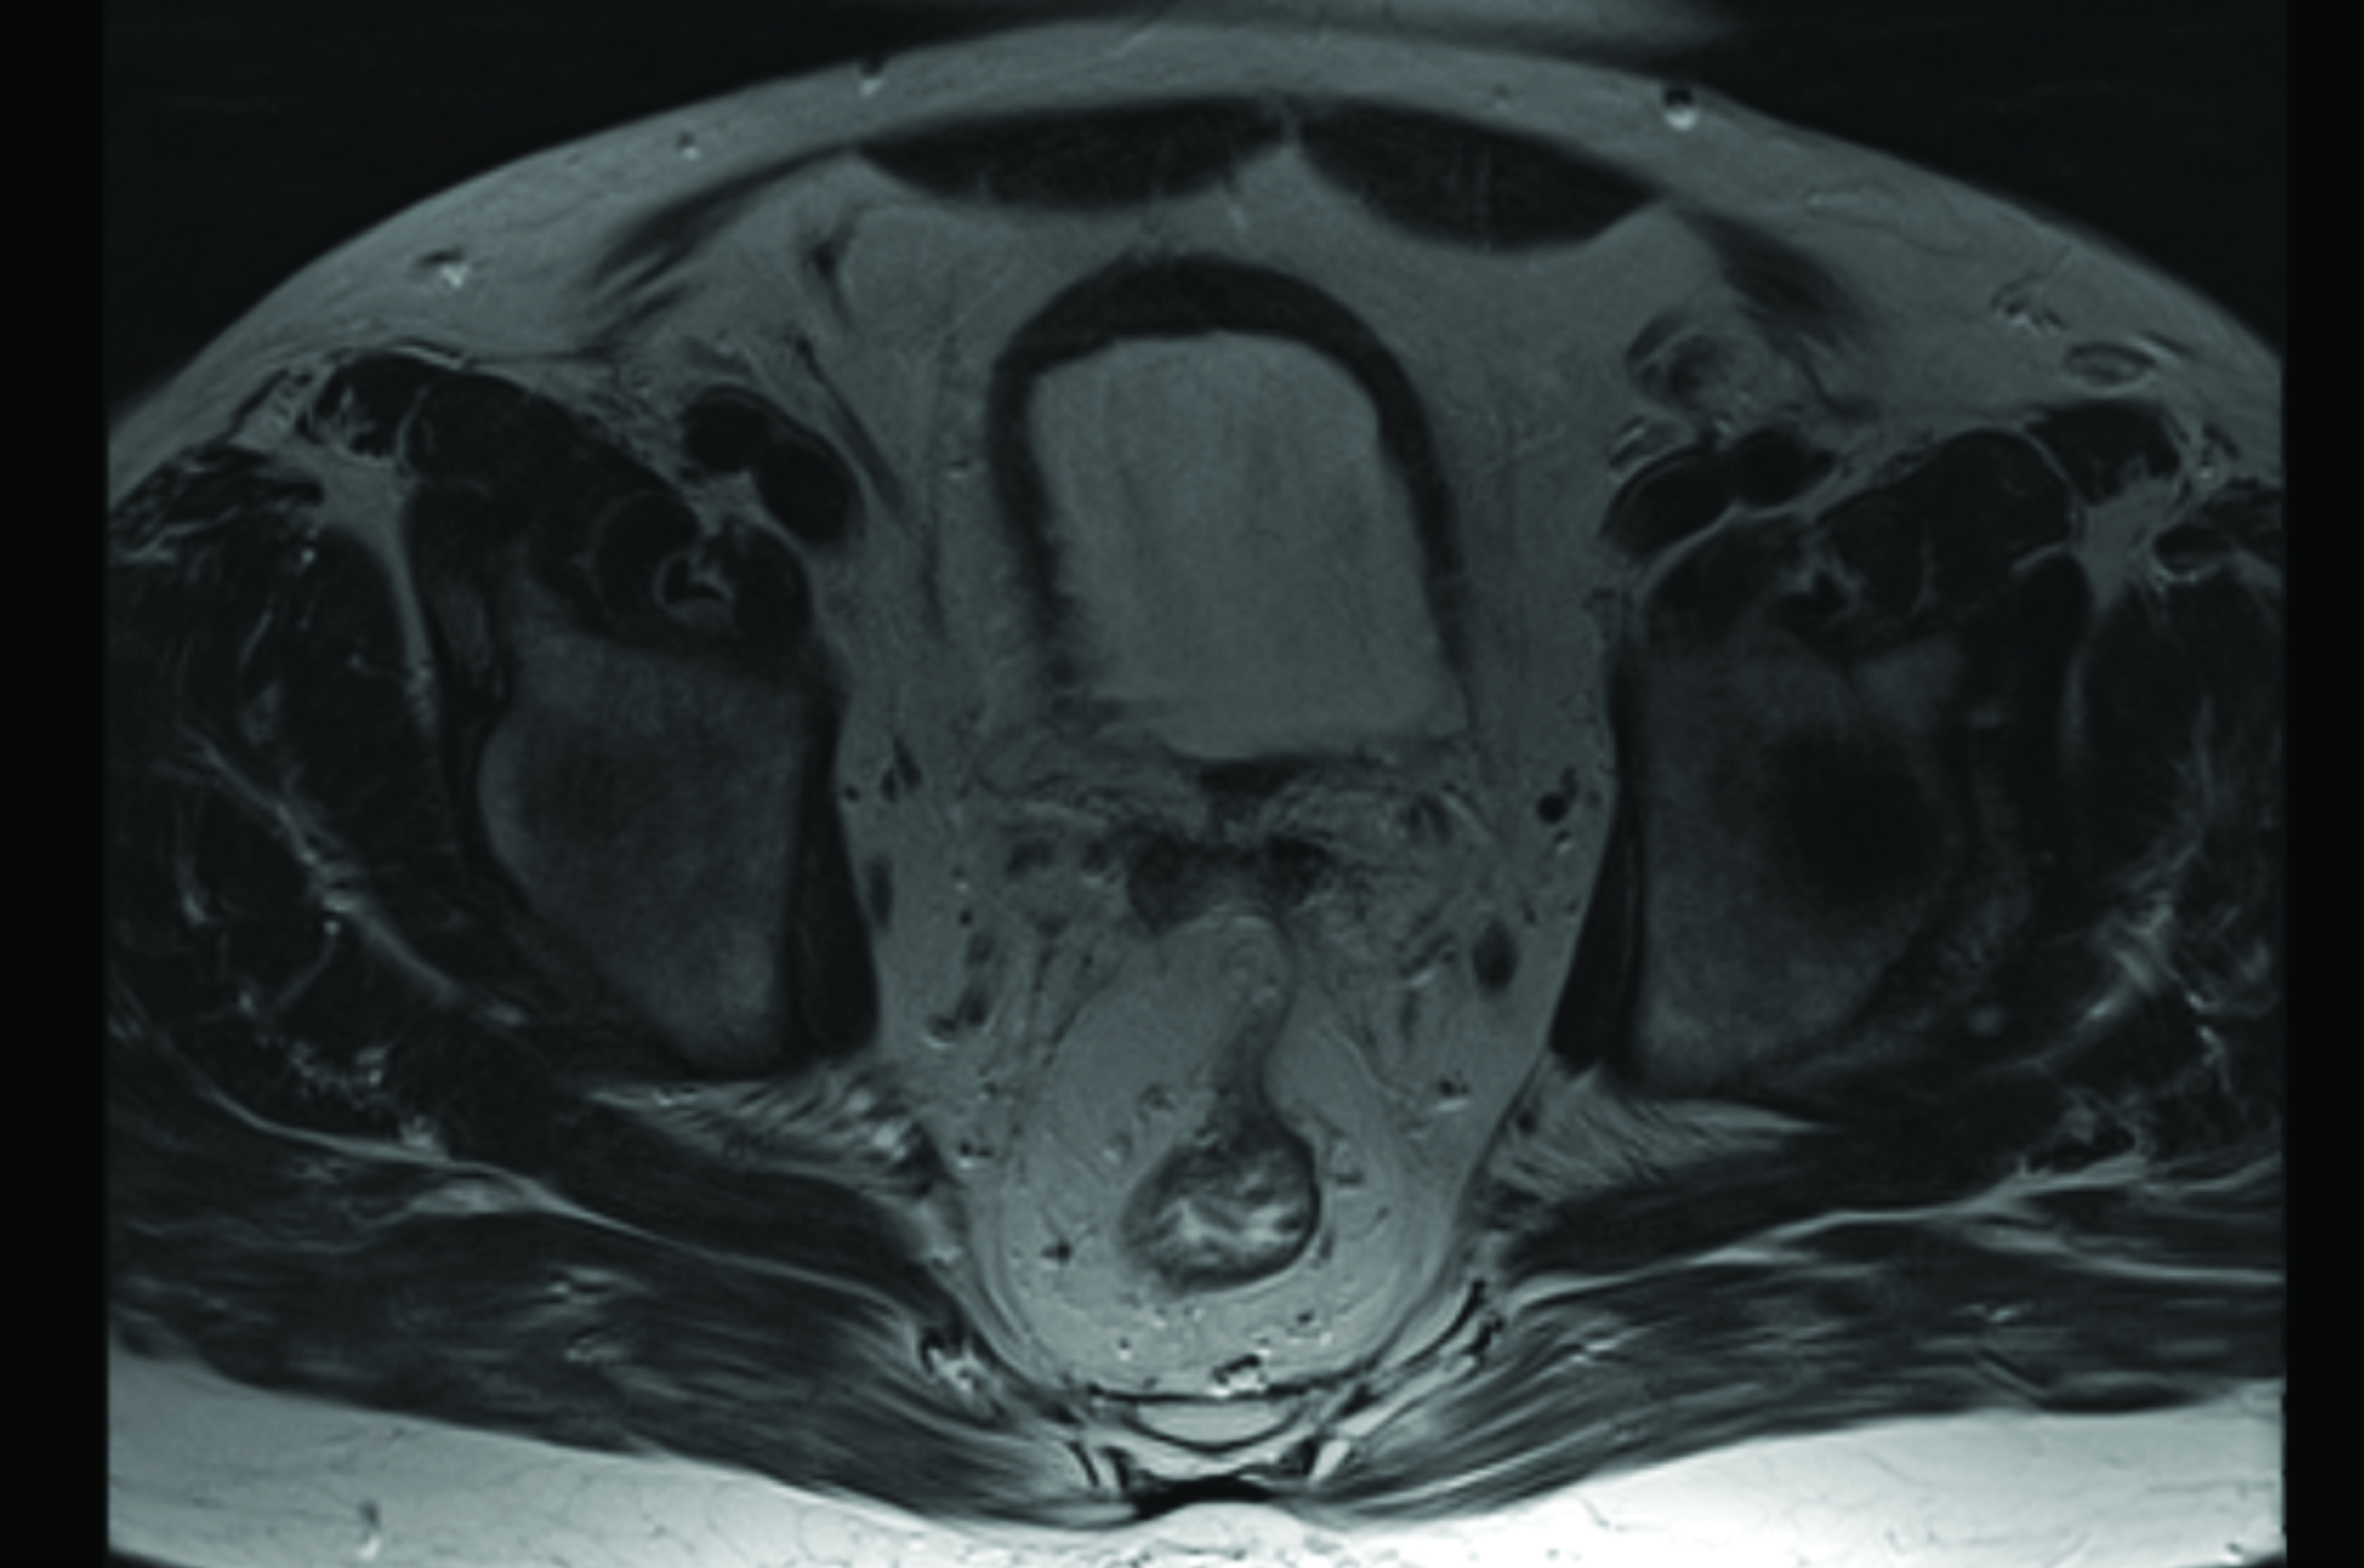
Pelvic MRI, performed after 12 months of androgen deprivation therapy, showing no suspect lymph node.
